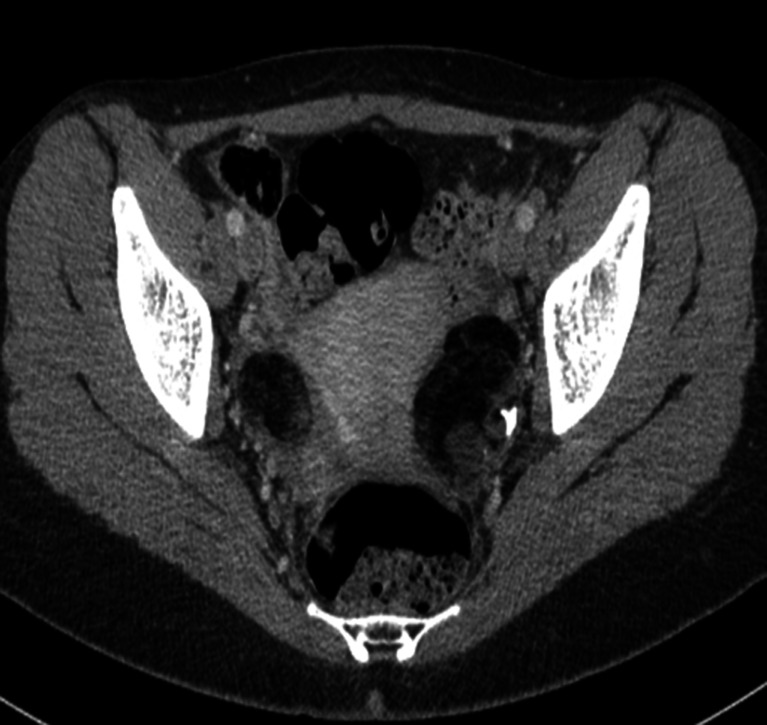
Case 2. Axial unenhanced CT scan demonstrates bilateral teratomas of the ovaries with intratumoral fat and focal calcifications (seen on the *left side*)
